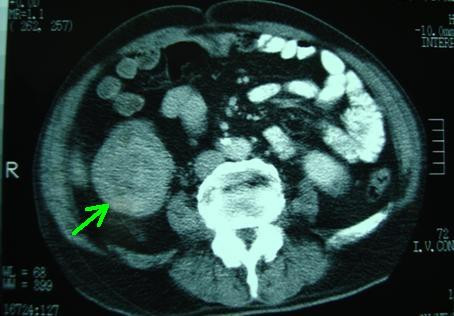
Computed tomography (CT) scan showing distension of the ascending colon with concomitant wall thickening and dimness of the pericolic fat tissue (green arrow).
